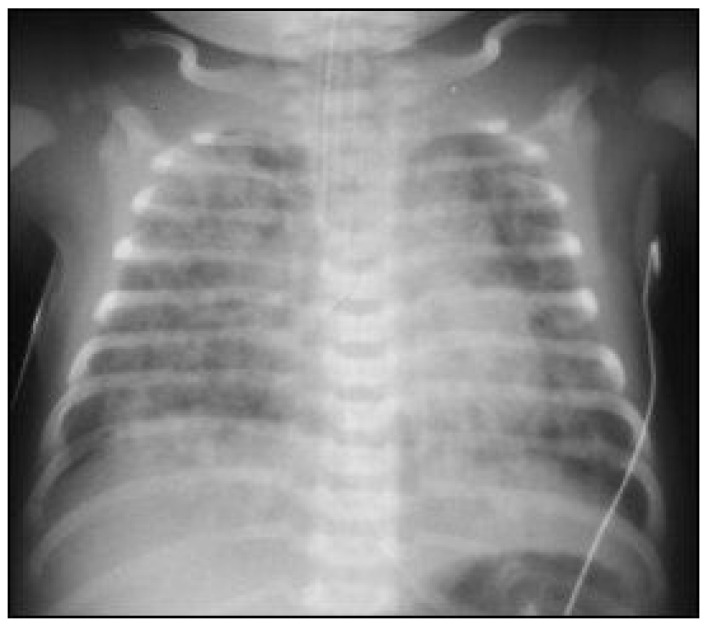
Chest radiograph demonstrating diffuse patchy opacification of the lungs, an example from an infant with meconium aspiration.
